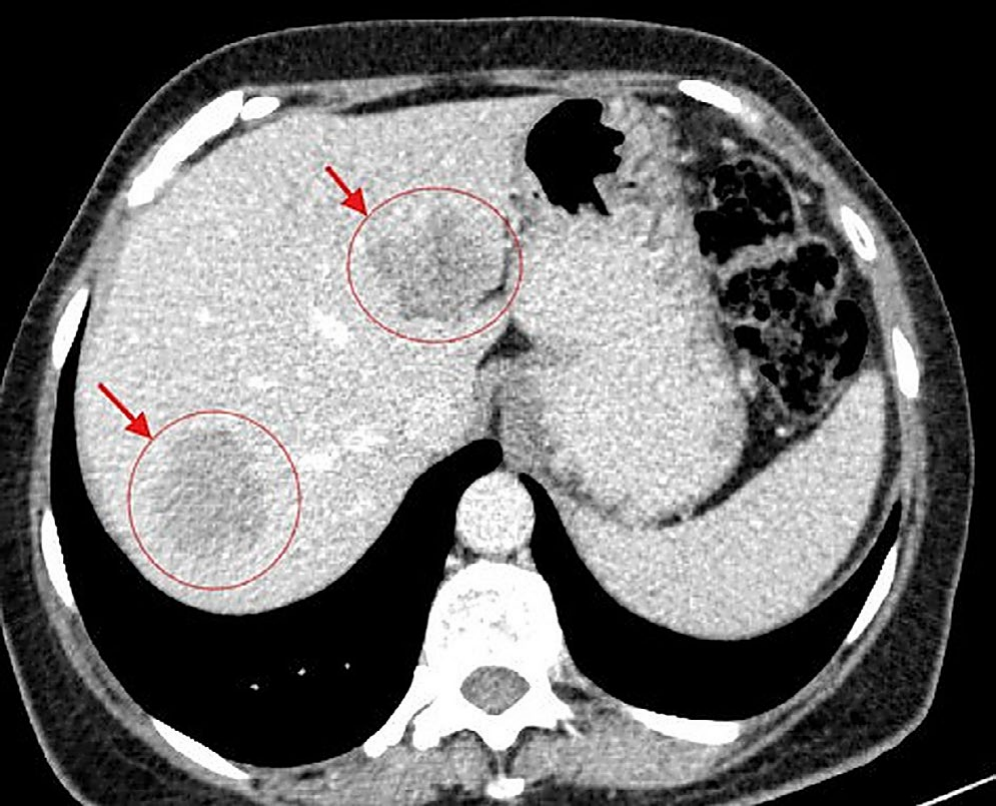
Baseline axial contrast‐enhanced computed tomography of the abdomen demonstrates hypodense lesions (red arrows) consistent with metastatic melanoma deposits involving both hepatic lobes.
